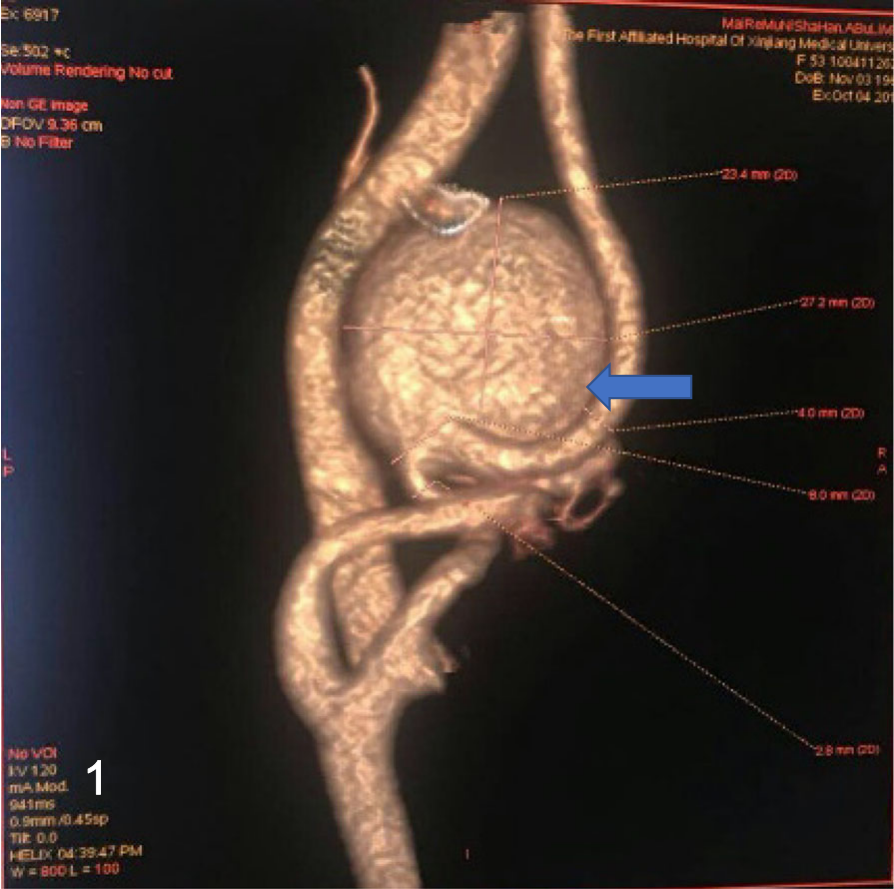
CTA showed the left EICA giant aneurysm (arrow), measuring the lesion was 27 × 23 mm in diameter
